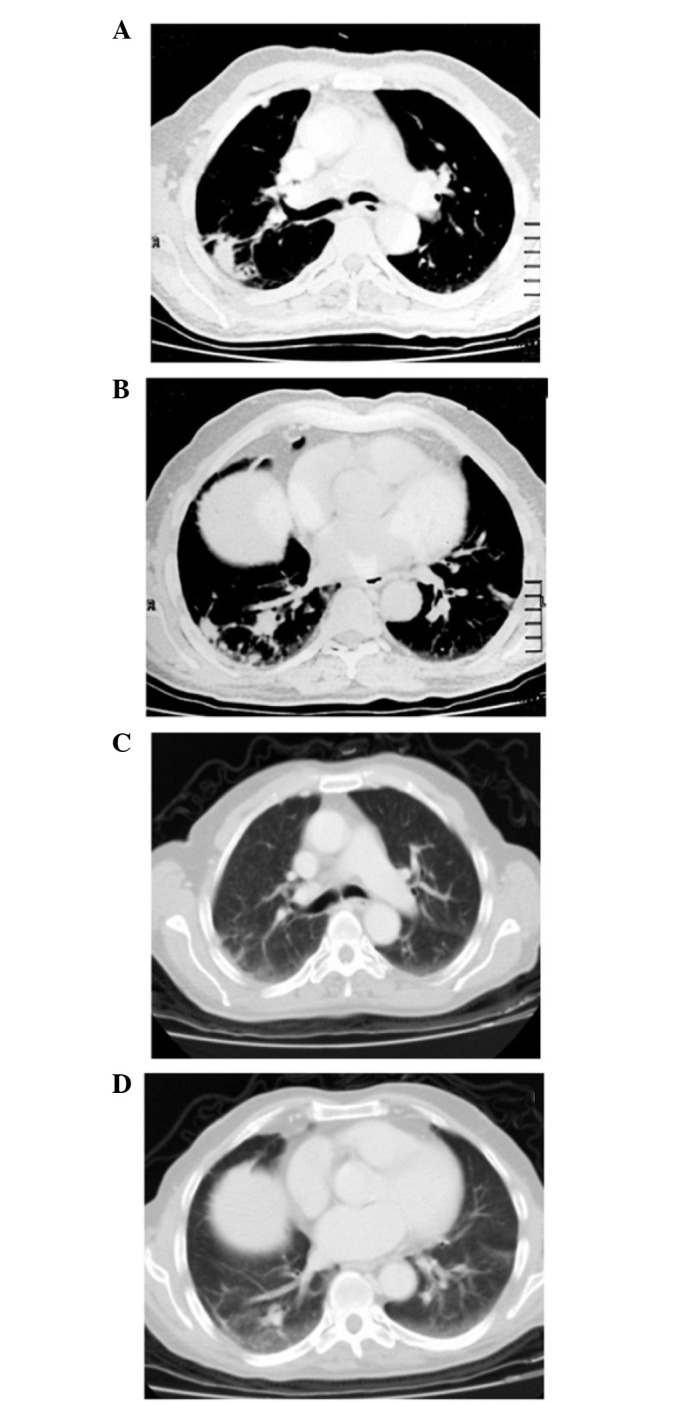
Initial chest computed tomography (CT) scans showing (A and B) multiple mediastinal lymph nodes.(at diagnosis the largest measured 12–13 mm in short-axis diameter) and multiple nodular lesions in the lung parenchyma, the largest measured 2×1.5 cm. (C and D) Follow-up chest CT scans following six courses of chemotherapy showing mediastinal lymph nodes with a short-axis diameter of <10 mm and segmental linear atelectasia in the parenchymal window, which is consistent with a complete response.
